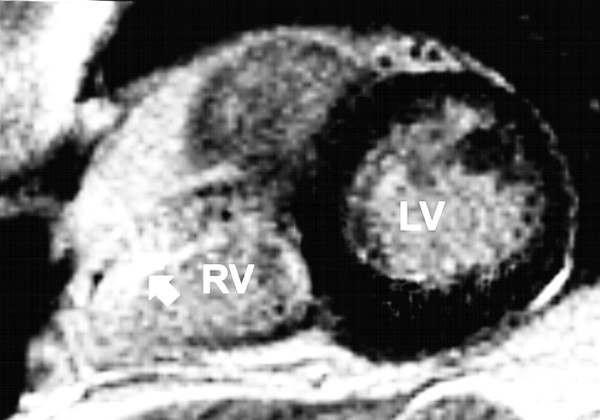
Magnetic resonance imaging of patient number 4 (short axis) featuring a homogenous pattern of the left ventricular myocardium (late enhancement, segmental inversion recovery - TurboFLASH 2D image). RV: right ventricle. LV: left ventricle. Arrow marks right ventricular late enhancement.
